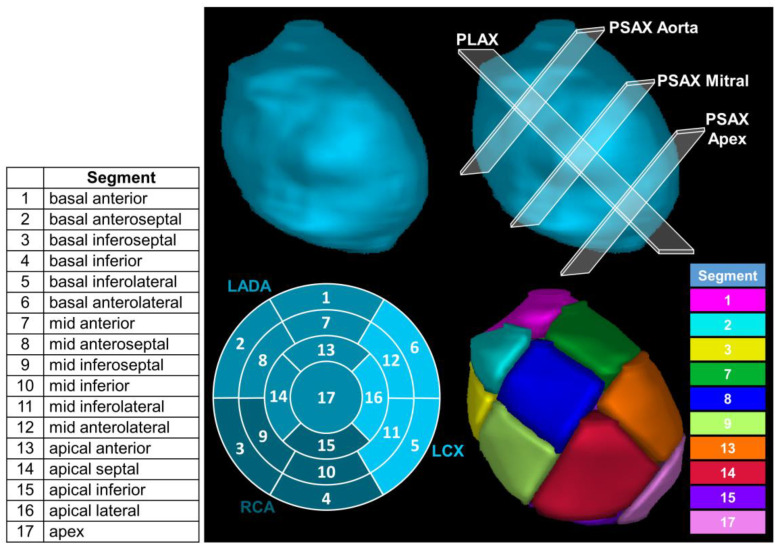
(Top left): Three-dimensional segmentation of left ventricle (LV). (Top right): The parasternal long-axis (PLAX) is the plane through the longest section of the LV and where the primary principal component goes through. The three parasternal short-axis (PSAX) planes were used to define the apex and three equal regions of equal length down the PLAX (i.e., the basal, mid-cavitary, and apical regions). (Bottom left): American Heart Association bulls eye displaying how segments are split. The three coronary artery territories are the left anterior descending artery (LADA), the right coronary artery (RCA), and the left circumflex coronary artery (LCX). (Bottom right): Display of the left ventricle split into 17 segments (color key denoting each displayed LV segment in the (bottom right)) based on 17-segment American Heart Association model.
